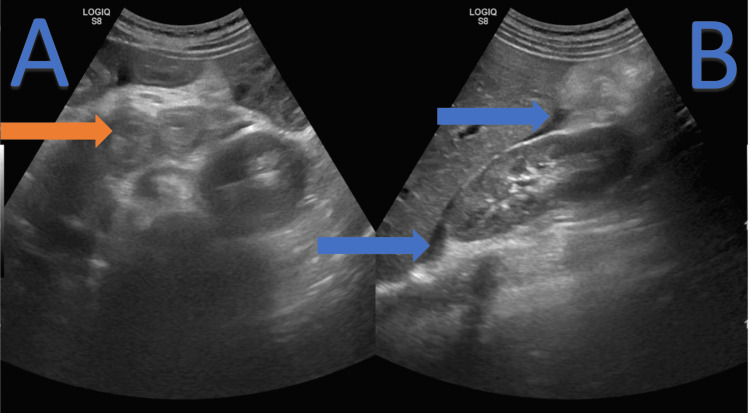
(A) Abdominal ultrasound (orange arrow) highlighting stratified parietal thickening involving the duodenum. (B) Blue arrows highlighting moderate ascites in Morrison space.
